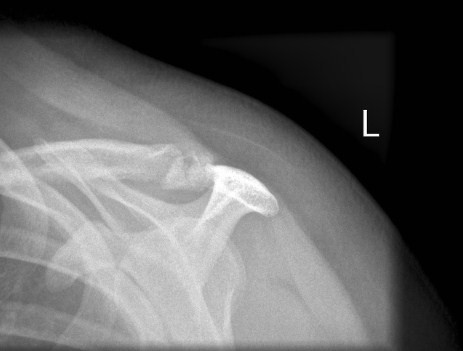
Pre-operative axial radiograph of the left shoulder. It shows an irregularity of the acromion which is poorly visualised. No double density sign is visible.
